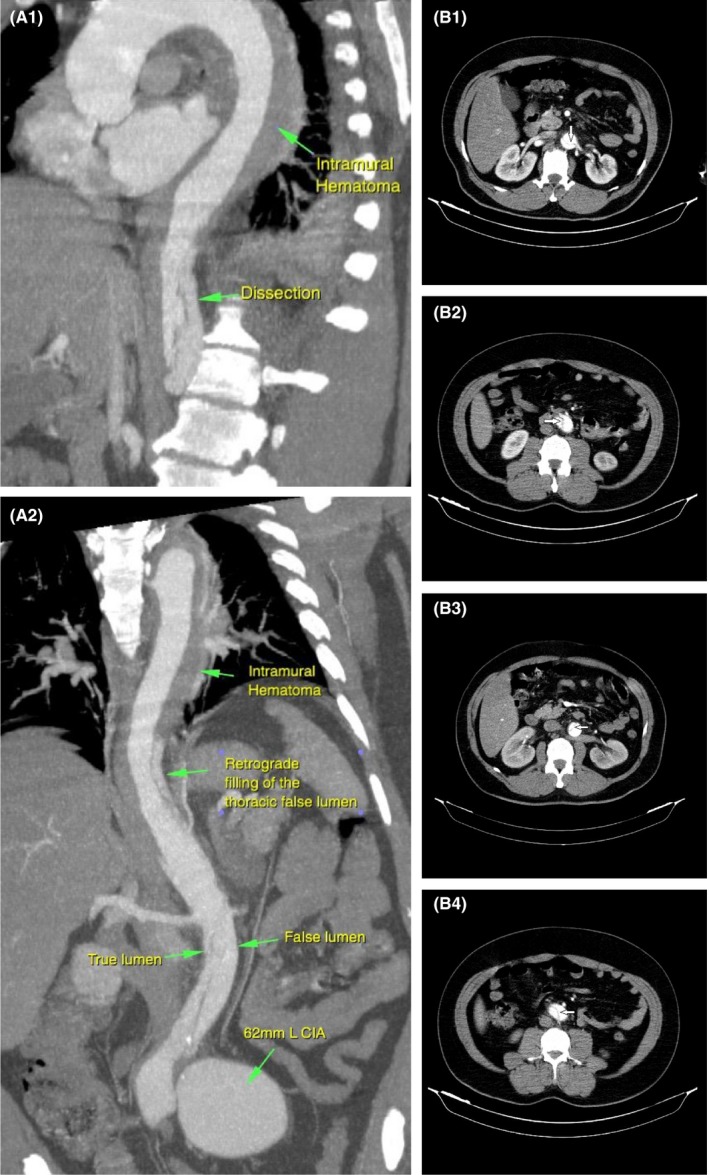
A, Aortic CT Angiography showing Type B aortic intramural hematoma & classic aortic dissection and left common iliac artery aneurysm. B, Aortic CT Angiography demonstrating Type B acute aortic dissection (arrows)
